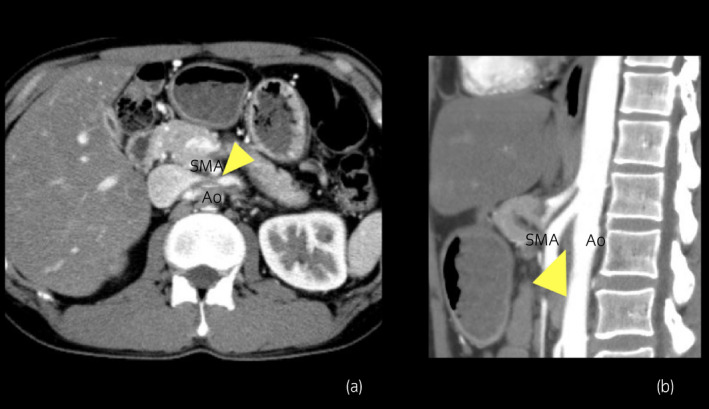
The CTA indicated that the distance between the aorta and SMA at the level of the LRV was 2.5 mm (arrow head) (a). The sagittal CTA showed that the angle between the Ao and SMA was 22° (arrow head) (b).
